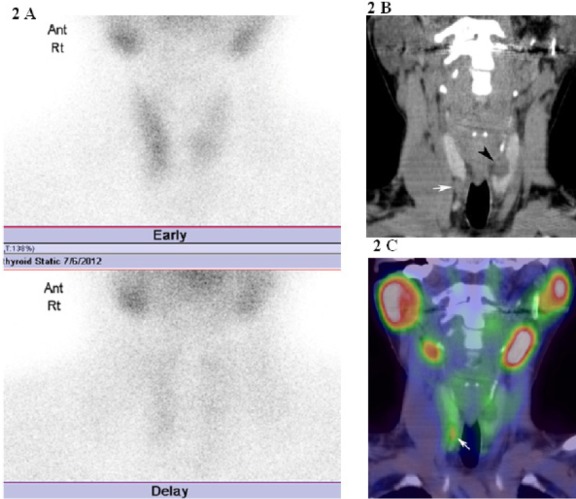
A. Initial and delayed phases of ^99m^ Tc-MIBI planar scintigraphy showed abnormal uptake. B. Coronal non-contrast enhanced CT of the neck. A lesion was detected in the inferior right portion of the thyroid lobe (arrow). A cystic mass was detected in the left lobe of the thyroid (arrowhead). C. A coronal fused image (SPECT/CT). The fusion image demonstrated a faint abnormal uptake of ^99m^Tc-MIBI in the inferior right portion of the thyroid lobe (arrow). Right inferior hyperplastic parathyroid gland was removed during surgery
